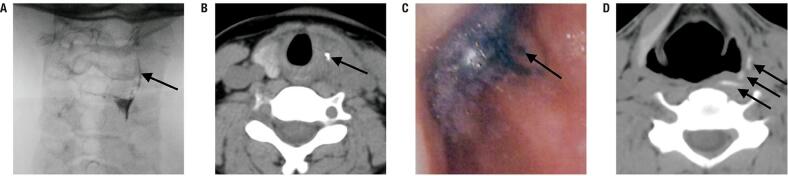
Diagnosis of PSF during the inflammatory stage. (A) Esophagram showing a fistula originating from the apex of the pyriform recess, and (B) CT scan showing the fistula filled with barium after esophagram (case 1). (C) Mythylene injected into the thyroid abscess and found by laryngoscopy (case 3). (D) CT scan performed 2 h after injection of meglumine diatrzoate into the thyroid abscess showing contrast agent infused into the piriform sinus (case 4).
